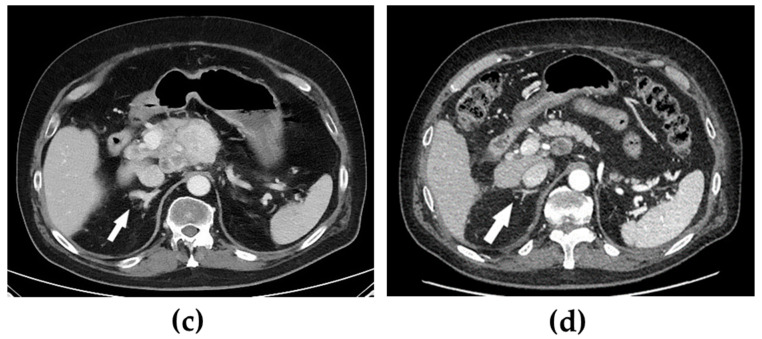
Computed tomography showing mediastinal and abdominal lymph node metastases before (a,c) and 12 months after (b,d) treatment with selpercatinib was started. Of additional note is a hyperplastic adrenal gland (arrows) before treatment that decreased in volume following treatment.
